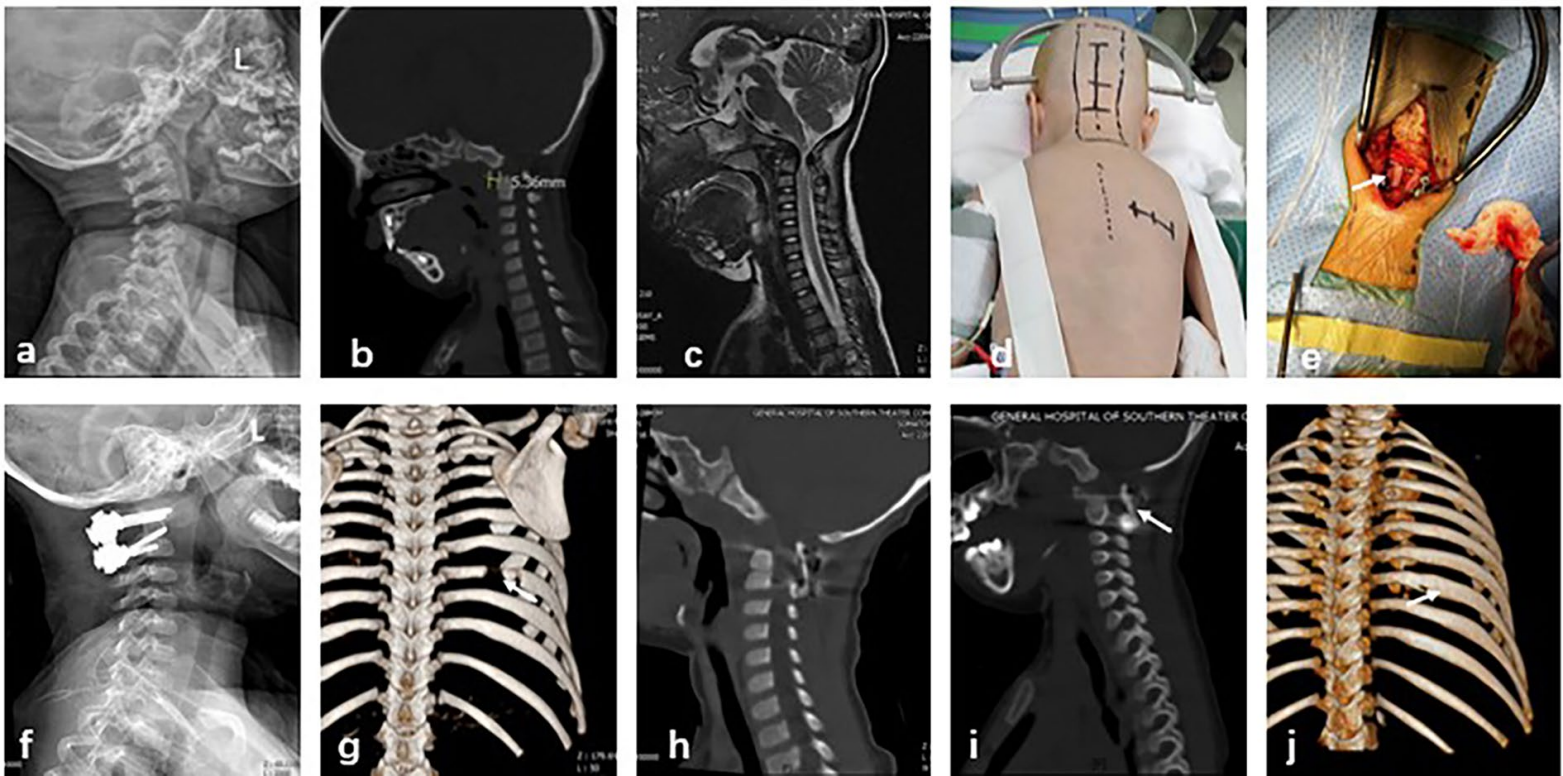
A 2-year-old female diagnosed with atlantoaxial dislocation. a–c: Preoperative X-ray, CT, and magnetic resonance imaging showing atlantoaxial dislocation, widening of the ADI, and spinal canal stenosis. d: Marking of the cervical incision site and oblique rib incision on the right side. e: Intraoperative use of autologous rib for structural bone grafting. f: Postoperative X-ray showing good positioning of the C1–C2 internal fixation. g: Chest CT 3D reconstruction at 1 week after surgery showing a defect in the right eighth rib. h: Sagittal CT of the cervical spine at 1 week after surgery showing good positioning of the rib bone graft. i: Follow-up cervical spine CT at 3 months after surgery showing bone fusion at the graft site. j: Chest CT 3D reconstruction at 3 months after surgery showing complete regeneration of the rib defect at the donor site
